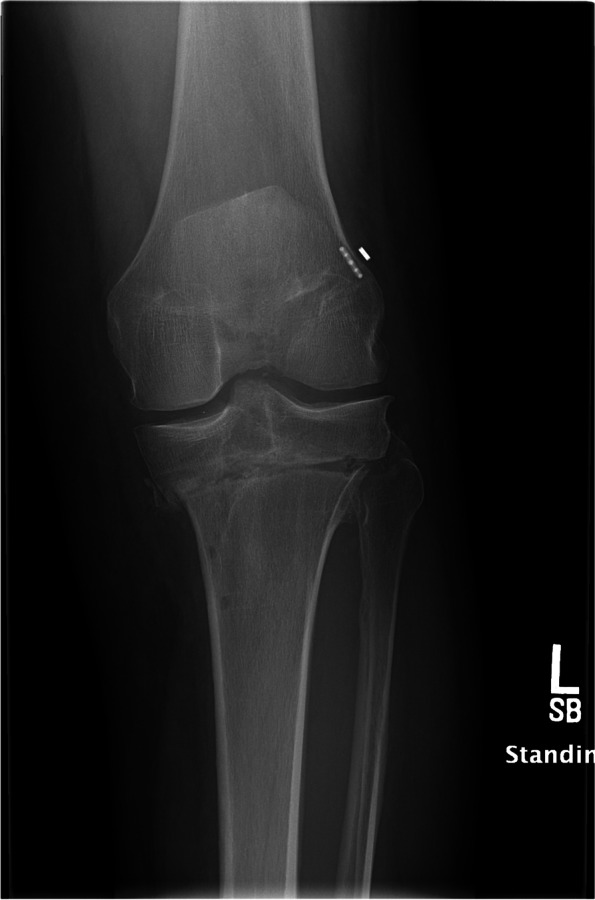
A 6-week post-operative radiograph demonstrating varus collapse of the osteotomy
